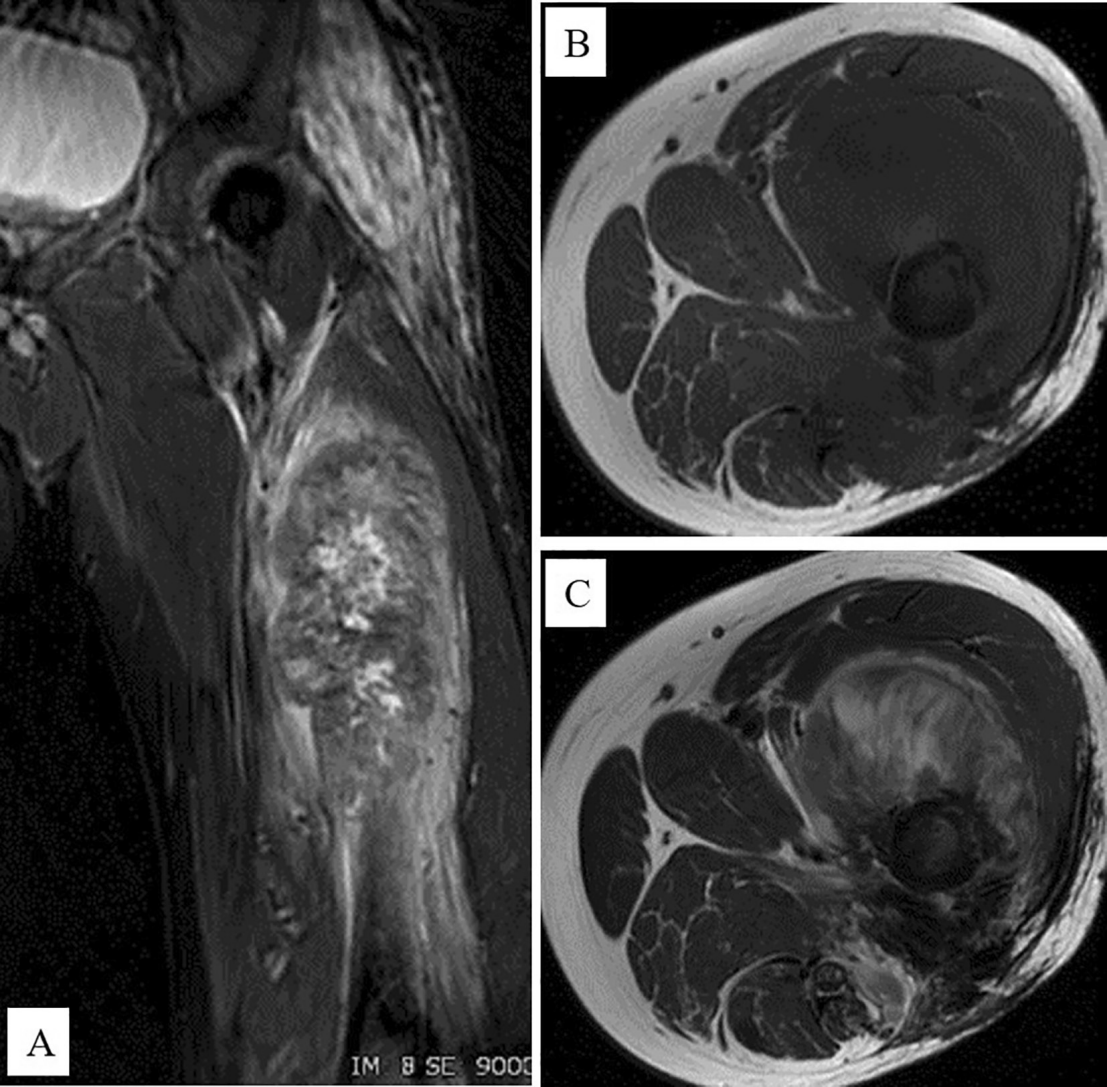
Magnetic resonance imaging analysis. Intramedullary tumor with low to intermediate and high signal on axial T2WI. Circumferential extra‐osseous tumor mostly shows a high signal, measuring 70 × 71 × 210 mm at 8 years after radiation. (A) Short TI inversion recovery coronal sequence. (B) T1WI axial sequence (femur muscle slice). (C) T2 WI axial sequence (femur muscle slice).
